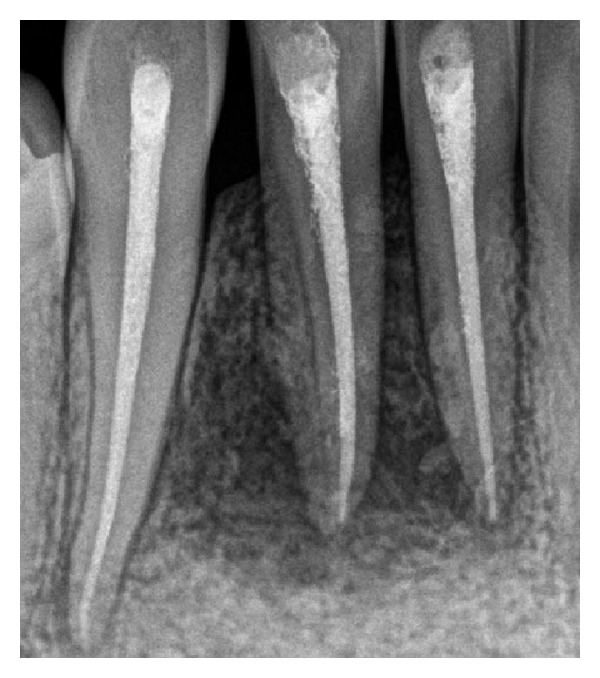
9 months postoperative radiograph.
